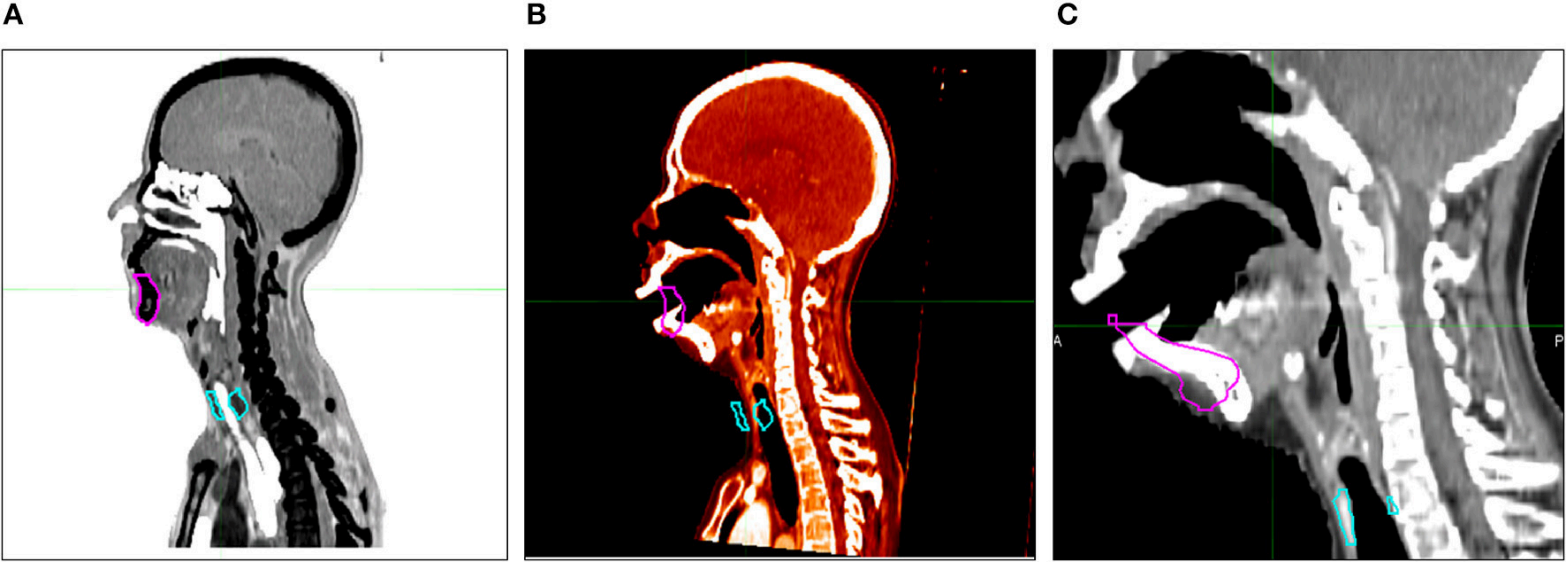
Auto-segmented contour results with open mouth target patient. (A) Atlas patient CT and its contour. (B) Target patient (open mouth) CT and selected atlas patient's contour. (C) Atlas segmentation-generated contour (AC).
